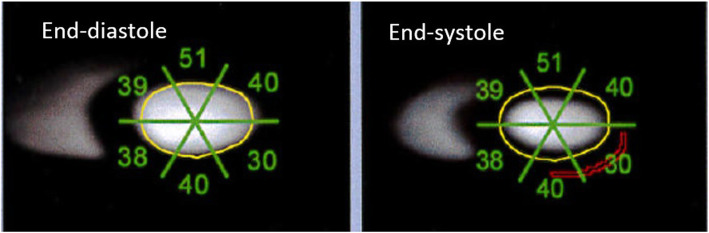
Automated segmentation of “pseudo-planar” PET/CT images summed along the cardiac short axis
